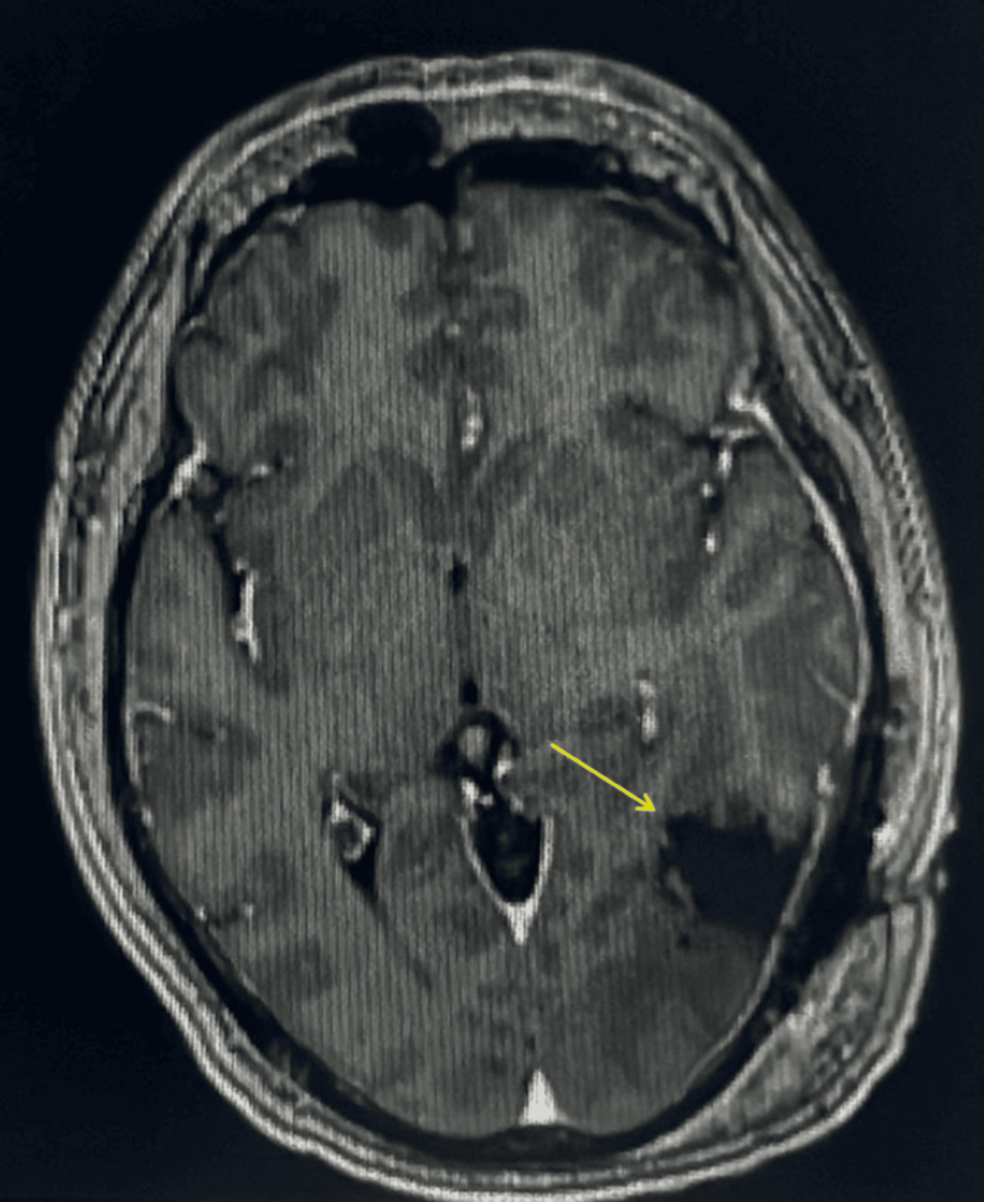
Post-operative brain MRI revealed an interval left temporal craniotomy (arrow) with a post-surgical cavity and no suspicious enhancement in the surgical area. MRI, magnetic resonance imaging
